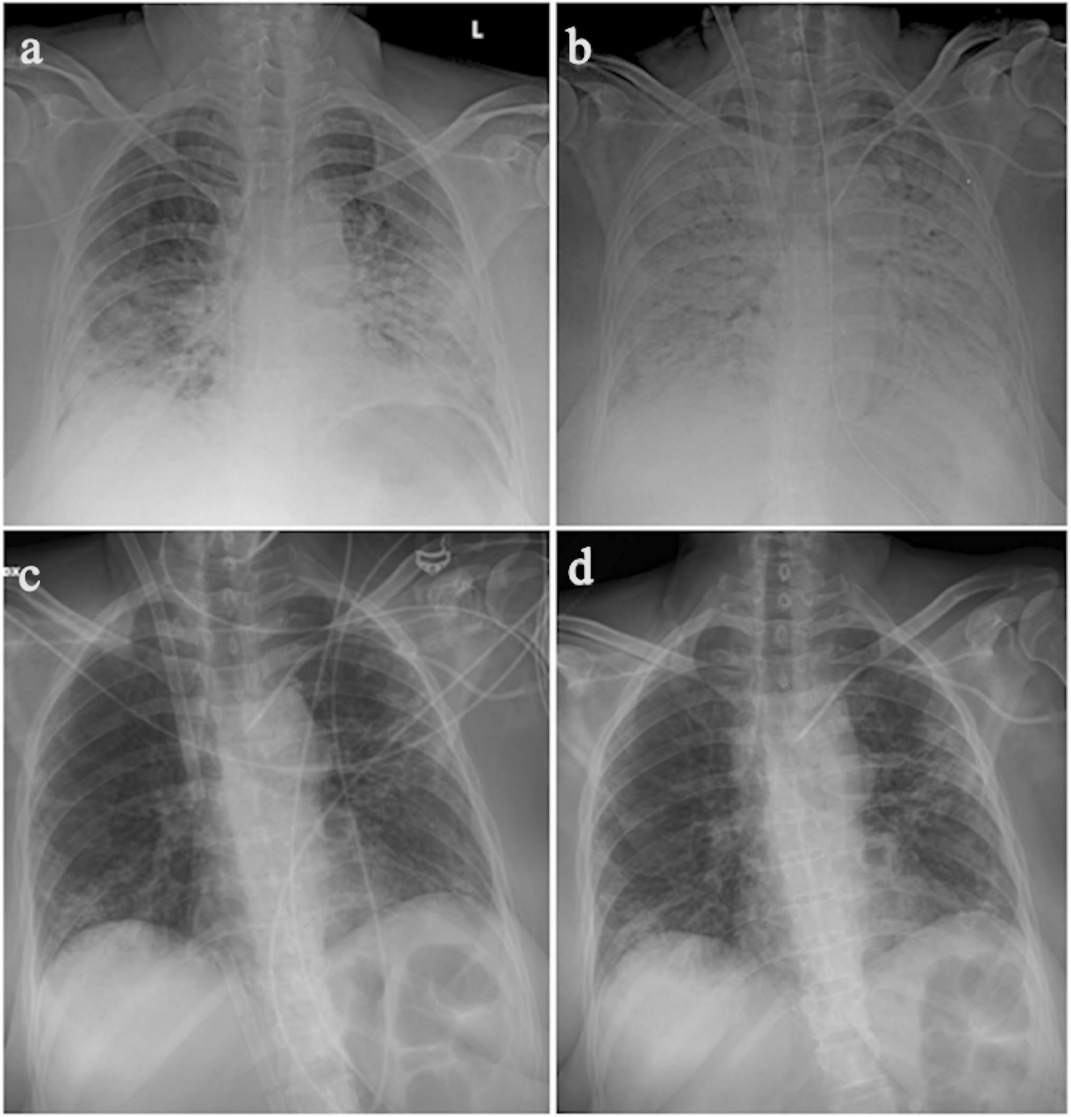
Chest radiographs a before and b after ECMO placement, showing correct placement of cannulae and complete whiteout of both lungs. c Chest radiographs performed at day 9 showing improvement in lung aeration and d) performed after ECMO removal, respectively
